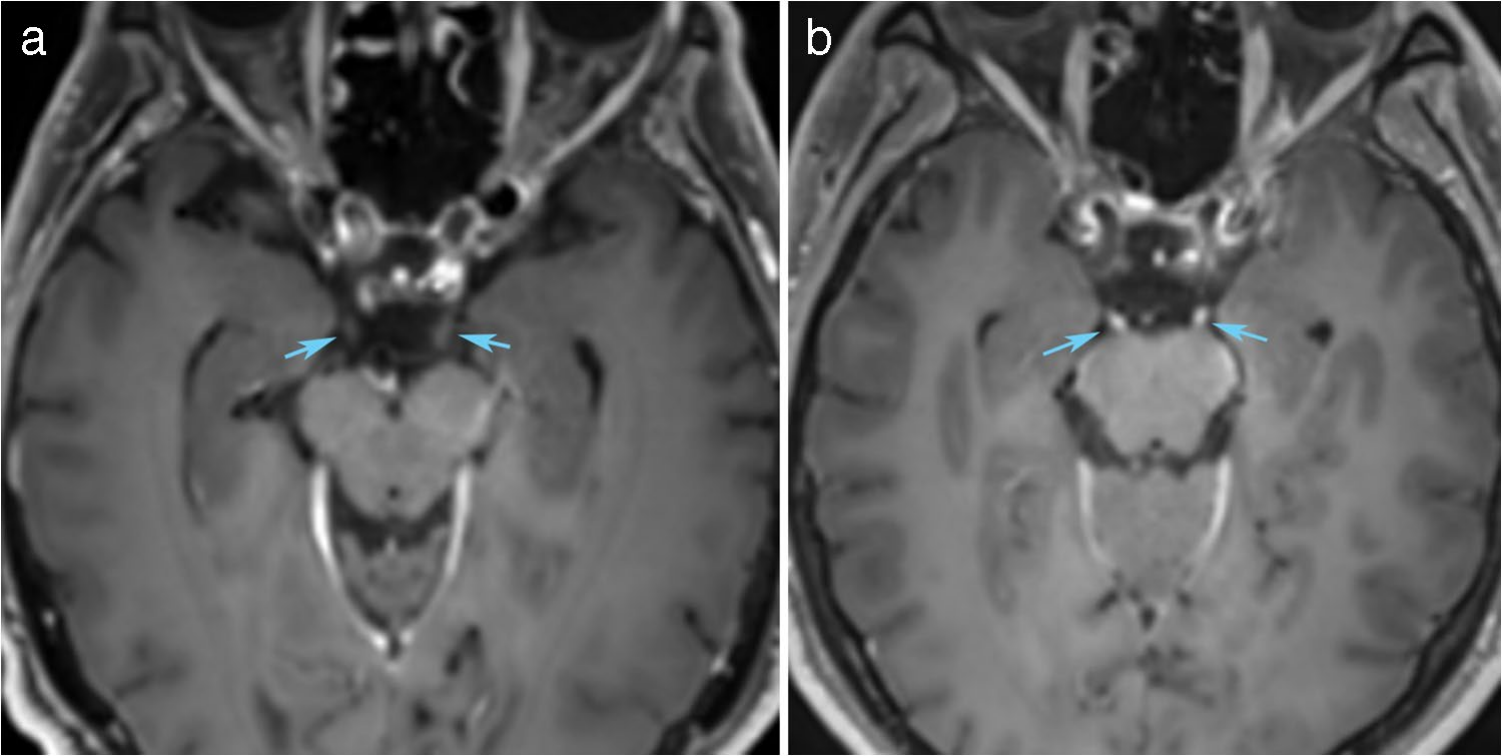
Demonstration of no versus strong enhancement of the oculomotor nerves on 1 mm axial image reconstruction from 3D T1-weighted and fat-suppressed acquisition. The subject in (a) demonstrates no enhancement of oculomotor nerves (arrows), while subject in (b) shows bilateral strong enhancement (arrows)
